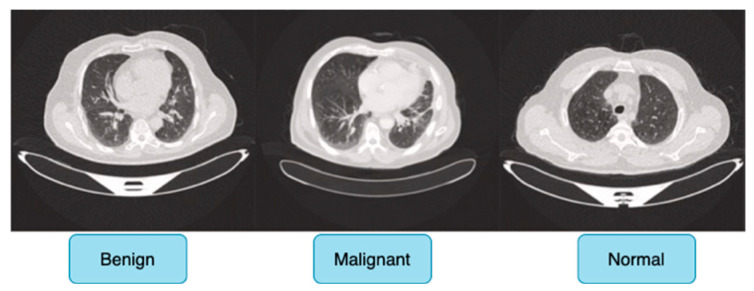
IQ-OTH/NCCD lung cancer CT dataset.
